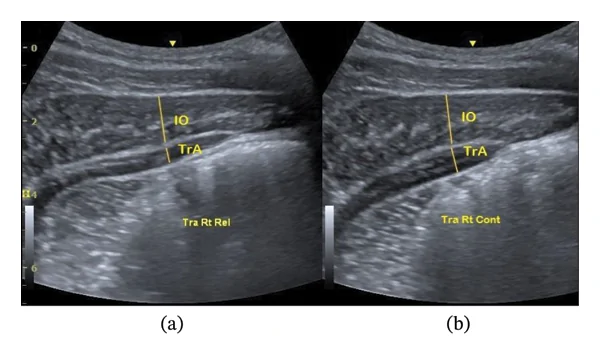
Ultrasound images of (a) relaxed internal oblique and transversus abdominis muscle thickness and (b) contracted internal oblique and transversus abdominis muscle thickness.
